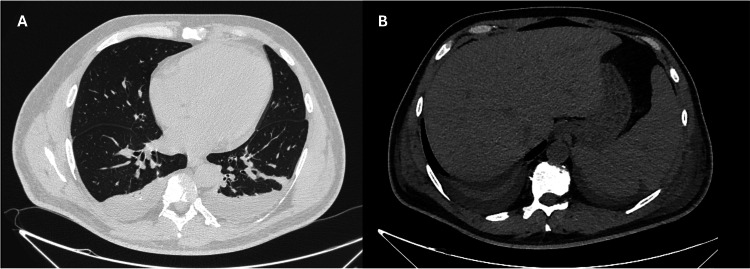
Computed tomography scan (A) The chest view shows bilateral pleural effusion. (B) The abdominal view shows perihepatic effusion, particularly in the subphrenic and paracolic regions. The pancreas is normal, showing no signs of inflammation, edema, or involvement in the pathological process.
